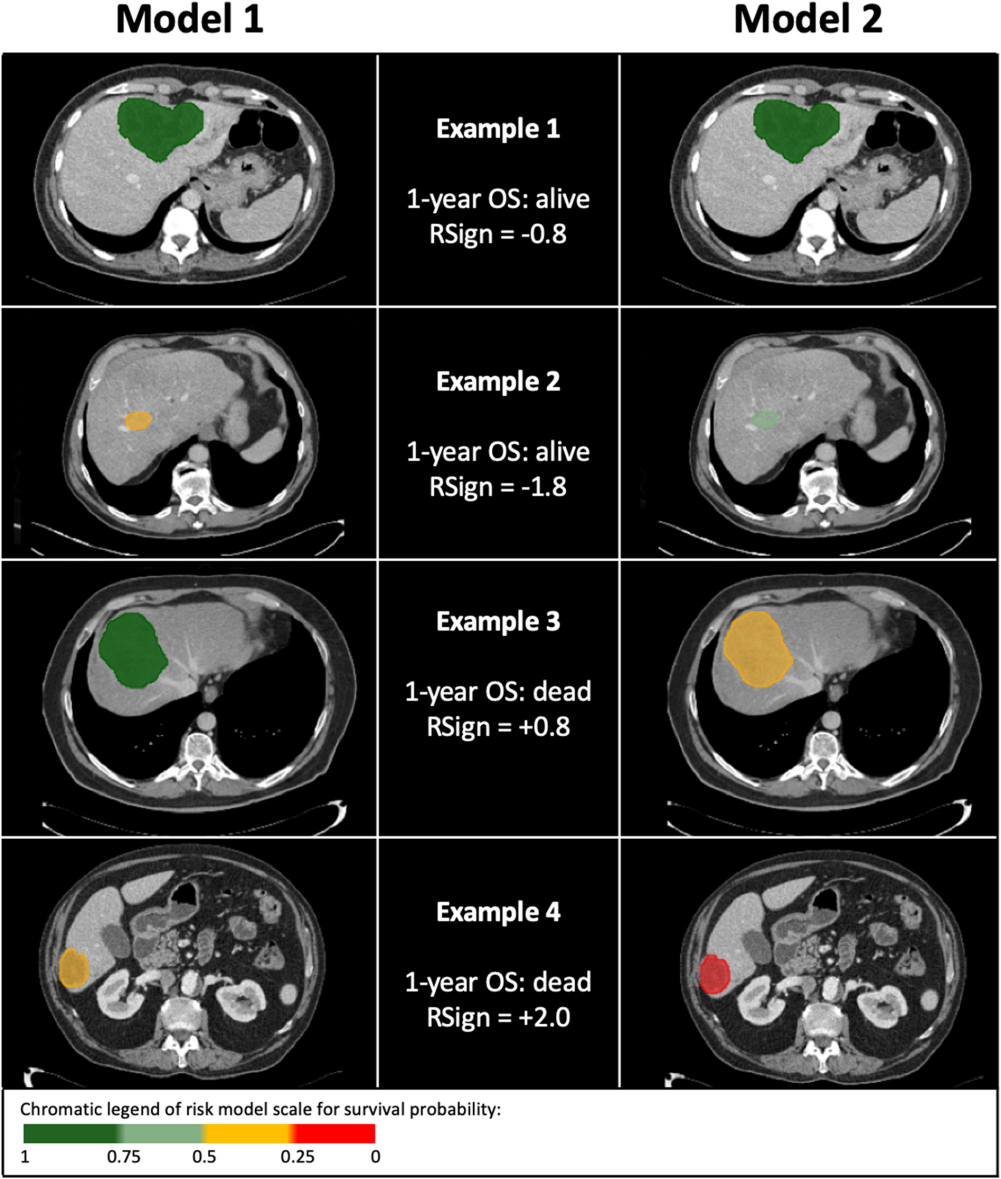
Chromatic representation of four examples with variable classification by either Model 1 or Model 2. Survival probability is reported in left column for Model 1 and right column for Model 2: the survival probability of each model is rendered by chromatic scale of the on tumor segmentation ROI (see chromatic legend in bottom box of the figure). The middle column details the outcome (alive or dead) of each case at 1 year since CT and the RSign value by reader 1. Example 1—large IMCC consistently classified with likelihood of 1-year survival > 0.75 by both Model 1 and Model 2 (RSign < 0.39), alive at 1 year. Example 2—small IMCC classified with likelihood of survival < 0.5 by Model 1 and likelihood of survival > 0.5 by Model 2 (RSign < 0.39), alive at 1 year. Example 3—large IMCC classified with high likelihood of survival > 0.75 by Model 1 and likelihood of survival < 0.5 by Model 2 (RSign > 0.39), dead at 1 year. Example 4—small IMCC classified with mid-low likelihood of survival < 0.5 by Model 1 and likelihood of survival < 0.25 by Model 2 (RSign > 0.39), dead at 1 year. Of note, example 2 and example 3 showed inconsistent risk stratification between Model 1 and Model 2. In these two cases, the inclusion of RSign (Model 2) improved the stratification of 1-year survival
